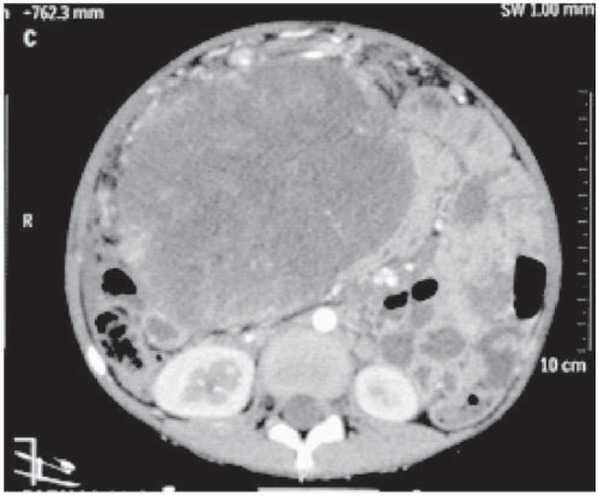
CT scan (axial section) showing the large solid cystic pelvic mass with septations.
